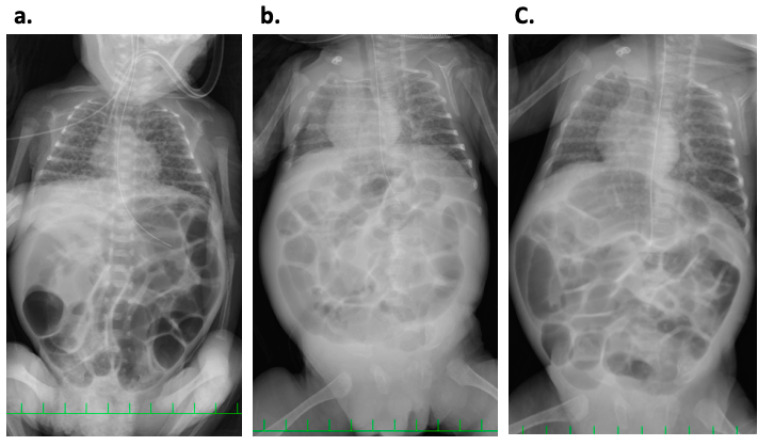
Abdominal X-ray of the case. Massive bowel dilatation was noted on (a) day 36, (b) day 85, and (c) day 98.
